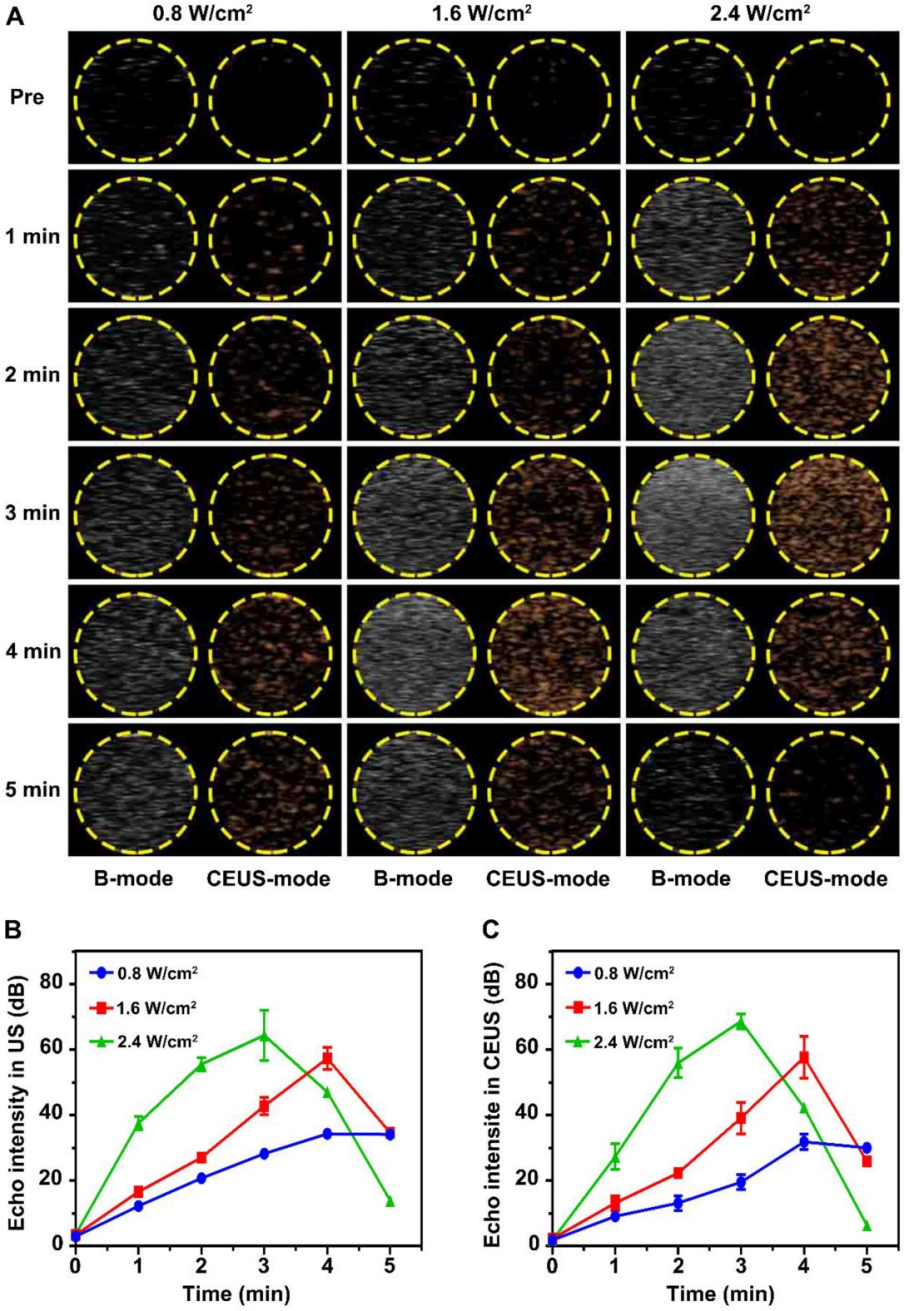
Liquid-gas phase transition using LIFU, and ultrasound imaging of Dex/PFP@LIPs-BMS-α *in vitro*. (A) B-mode and CEUS-mode ultrasound imaging of Dex/PFP@LIPs-BMS-α after being irradiated by LIFU at different points in time (pre, 1-5 min) and acoustic strength (0.8, 1.6 and 2.4 W/cm^2^). Quantitative analysis of signal intensity in B-mode (B) and CEUS-mode (C). Data are presented as mean ± SD.
